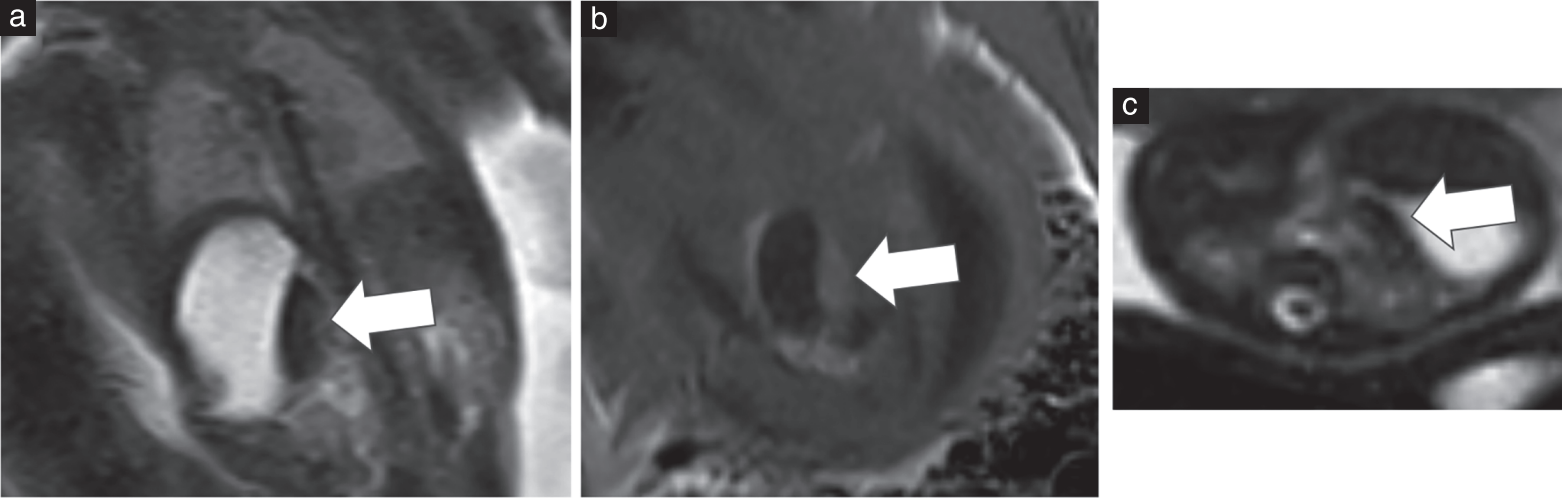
Coronal (a,b) and axial (c) magnetic resonance images of the fetal liver. ‘Hook‐shaped’ portion of liver parenchyma derived from left liver lobe appeared hypointense on T2‐weighted imaging (arrow) (a,c) and hyperintense on T1‐weighted imaging (arrow) (b). The abnormal liver segment extended towards the small curvature of the stomach.
